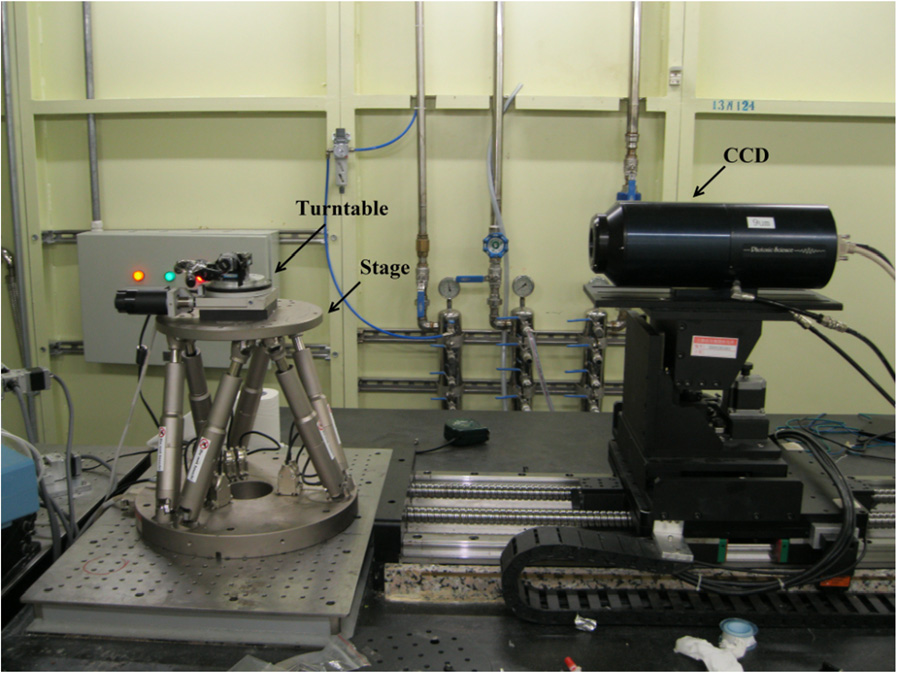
Setup of the X-ray Imaging and biomedical application beamline unit, Shanghai Synchrotron Radiation Facility. The stage has a six-joint control system that allows placement of the sample in the center of the field of view of the CCD. During scanning, the sample is placed on the turntable and the turntable is rotated around its cylindrical axis by 180° in steps of 1°.
